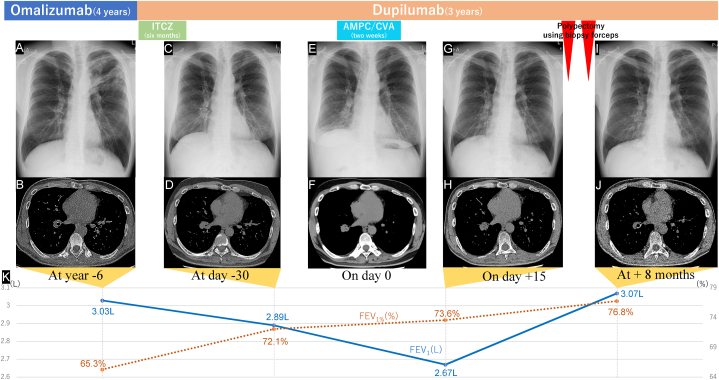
Clinical course on chest images and PFT (A) Chest radiograph at year −6 shows ill-defined consolidation, nodules, and opacities along the bronchus in the left upper lobe, accompanied by bronchial wall thickening. (B) Chest CT at year −6 shows no apparent intratracheal lesions in the right lower lung lobe. (C) Chest radiograph at day −30 shows significant improvement in the consolidation in the left upper lung lobe. However, irregular opacities around the truncus intermedius and elevation of the diaphragm on the right side are observed. (D) Chest CT at day −30 shows constriction of the lumen of the truncus basalis. (E) Chest radiograph on day 0 shows right basilar opacities with volume loss and further elevation of the diaphragm on the right side. (F) Chest CT on day 0 shows complete occlusion of the truncus basalis with a low-density area containing mucus at the center of the occluded lesion. (G) Chest radiograph on day +15 shows improved right basilar opacities and a partial reduction in the diaphragm elevation on the right side. (H) Chest CT on day +15 shows an endobronchial soft tissue nodule in the truncus basalis without a low-density area. (I) Chest radiograph at + 8 months shows no new lesions. Although linear and reticular shadows persist, the right lower lobe bronchus is preserved. (J) Chest CT at + 8 months shows complete resolution of the obstruction in the truncus basalis of the right bronchial lumen. (K) PFTs conducted two months after omalizumab initiation reveal an FEV1% of 65.3%, with an FEV1 of 3.03 L. On day -30, PFTs reveal an FEV1% of 72.1% and an FEV1 of 2.89 (L) On day +15, FEV1% and FEV1 are 73.6% and 2.67 L, respectively. At +8 months, the FEV1% and FEV1 values have improved to 76.8% and 3.07 L, respectively. Abbreviations: CT, computed tomography; PFT, pulmonary function test; ITCZ, itraconazole; AMPC/CVA, amoxicillin-clavulanate; FEV1%, forced expiratory volume in one second as a percent of forced vital capacity; FEV1, forced expiratory volume in one second.
